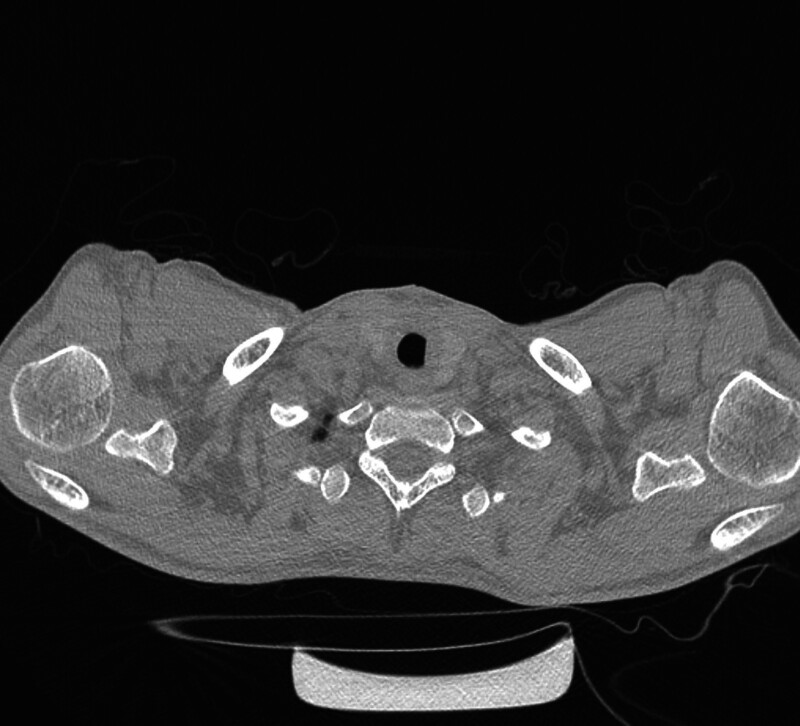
CT image of trachea on the 10th postoperative day. CT = computed tomography.
